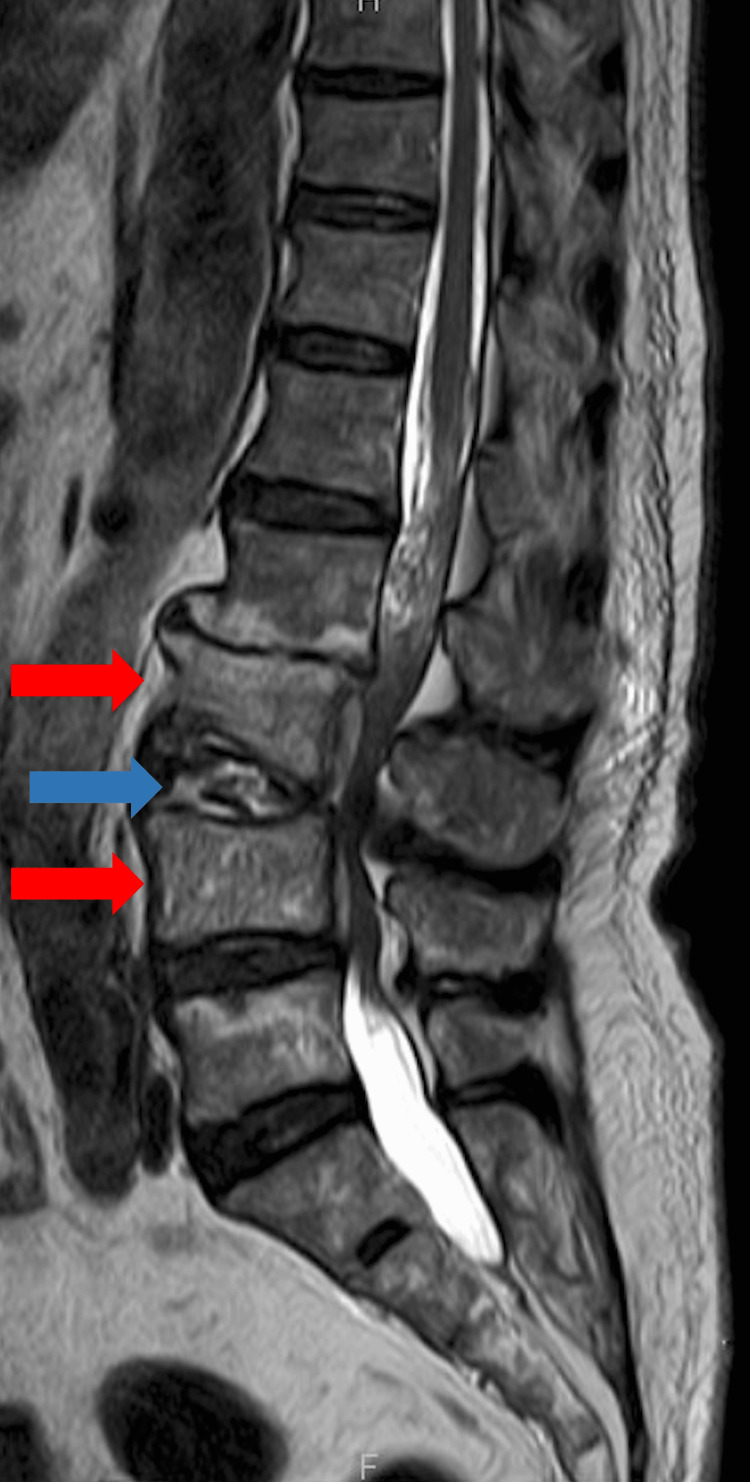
Magnetic resonance imaging of the lumbar spine on day nine showed hyperintensity of the L3 and L4 vertebral bodies (red arrows) and the contiguous disk (blue arrow).
